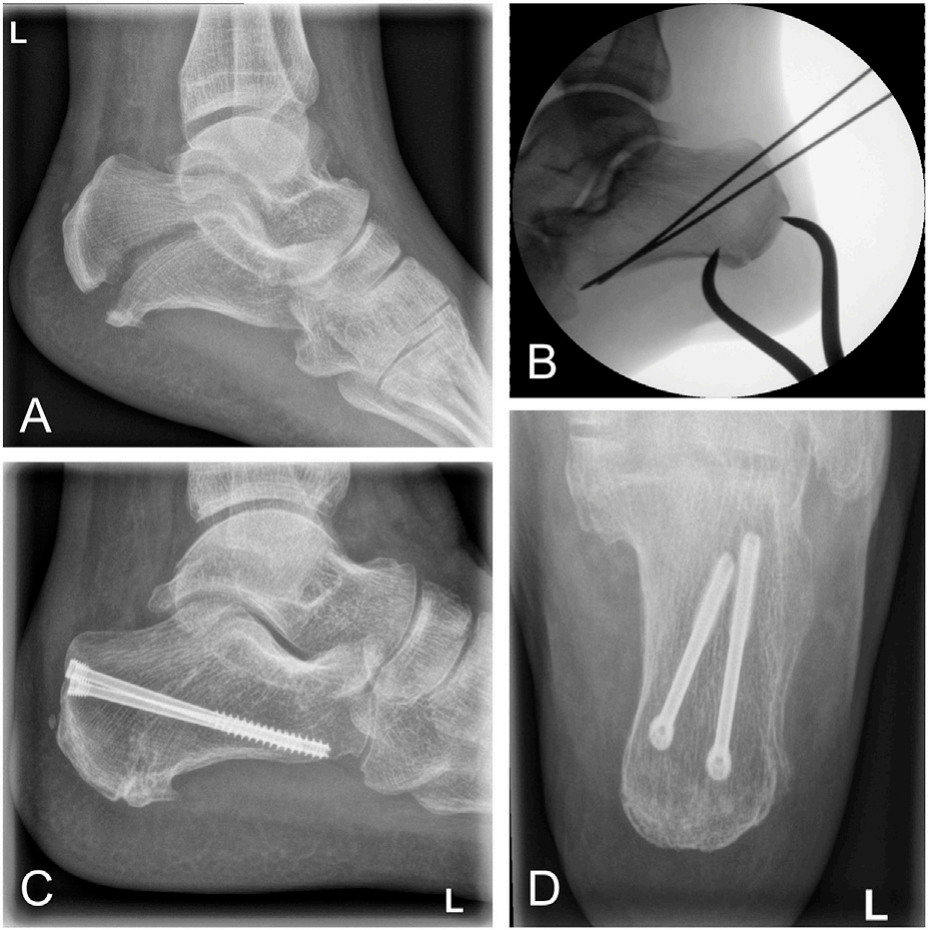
Clinical case of a calcaneal avulsion fracture successfully treated using 5.0-mm headless cannulated compression screws. (A) Lateral x-ray showing a fracture gap caused by tension of the Achilles tendon. (B) Intraoperative fluoroscopy demonstrating percutaneous reduction by a pointed reduction clamp. (C and D) Postoperative x-ray after 12 months demonstrating osseous healing in lateral and ap views.
